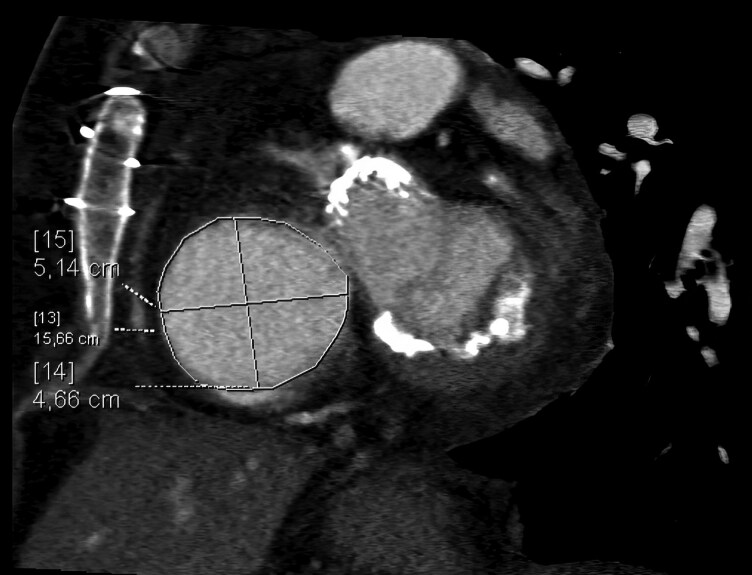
CT assessment of the tricuspid valve annulus with corresponding measurements.
